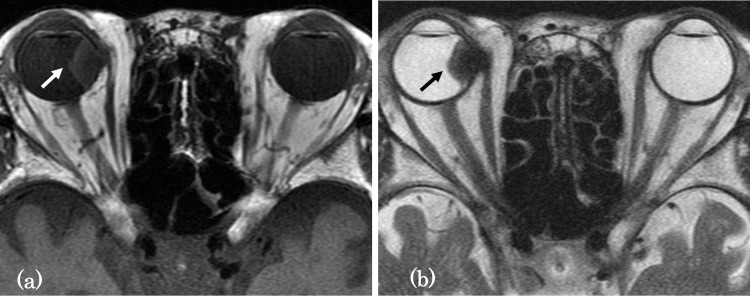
Axial MR images T1-weighted image (a) shows a choroidal mass with homogeneously isointense signal intensity compared with the cerebral cortex (white arrow). T2-weighted image (b) reveals that the mass is heterogeneous, mixed with hypointense and hyperintense signal intensities relative to the cerebral cortex (black arrow).
